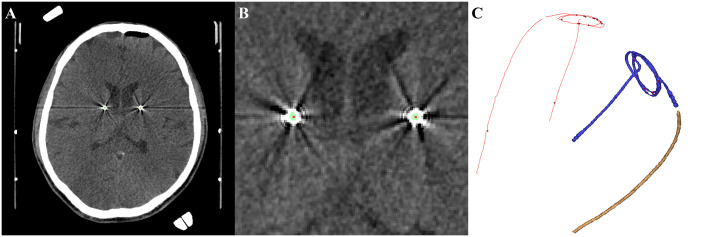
Process of 3-dimensional reconstruction and centerline rendering. A. A postoperative brain computed tomography scan was used in the calculation. B. Green indicates intracranial leads, which were defined as an area of Hounsfield units exceeding 2700, and red indicates the centerline rendered with software logic. C. Reconstructed intracranial lead (right side, volume rendered) and rendered centerline (left side, red).
